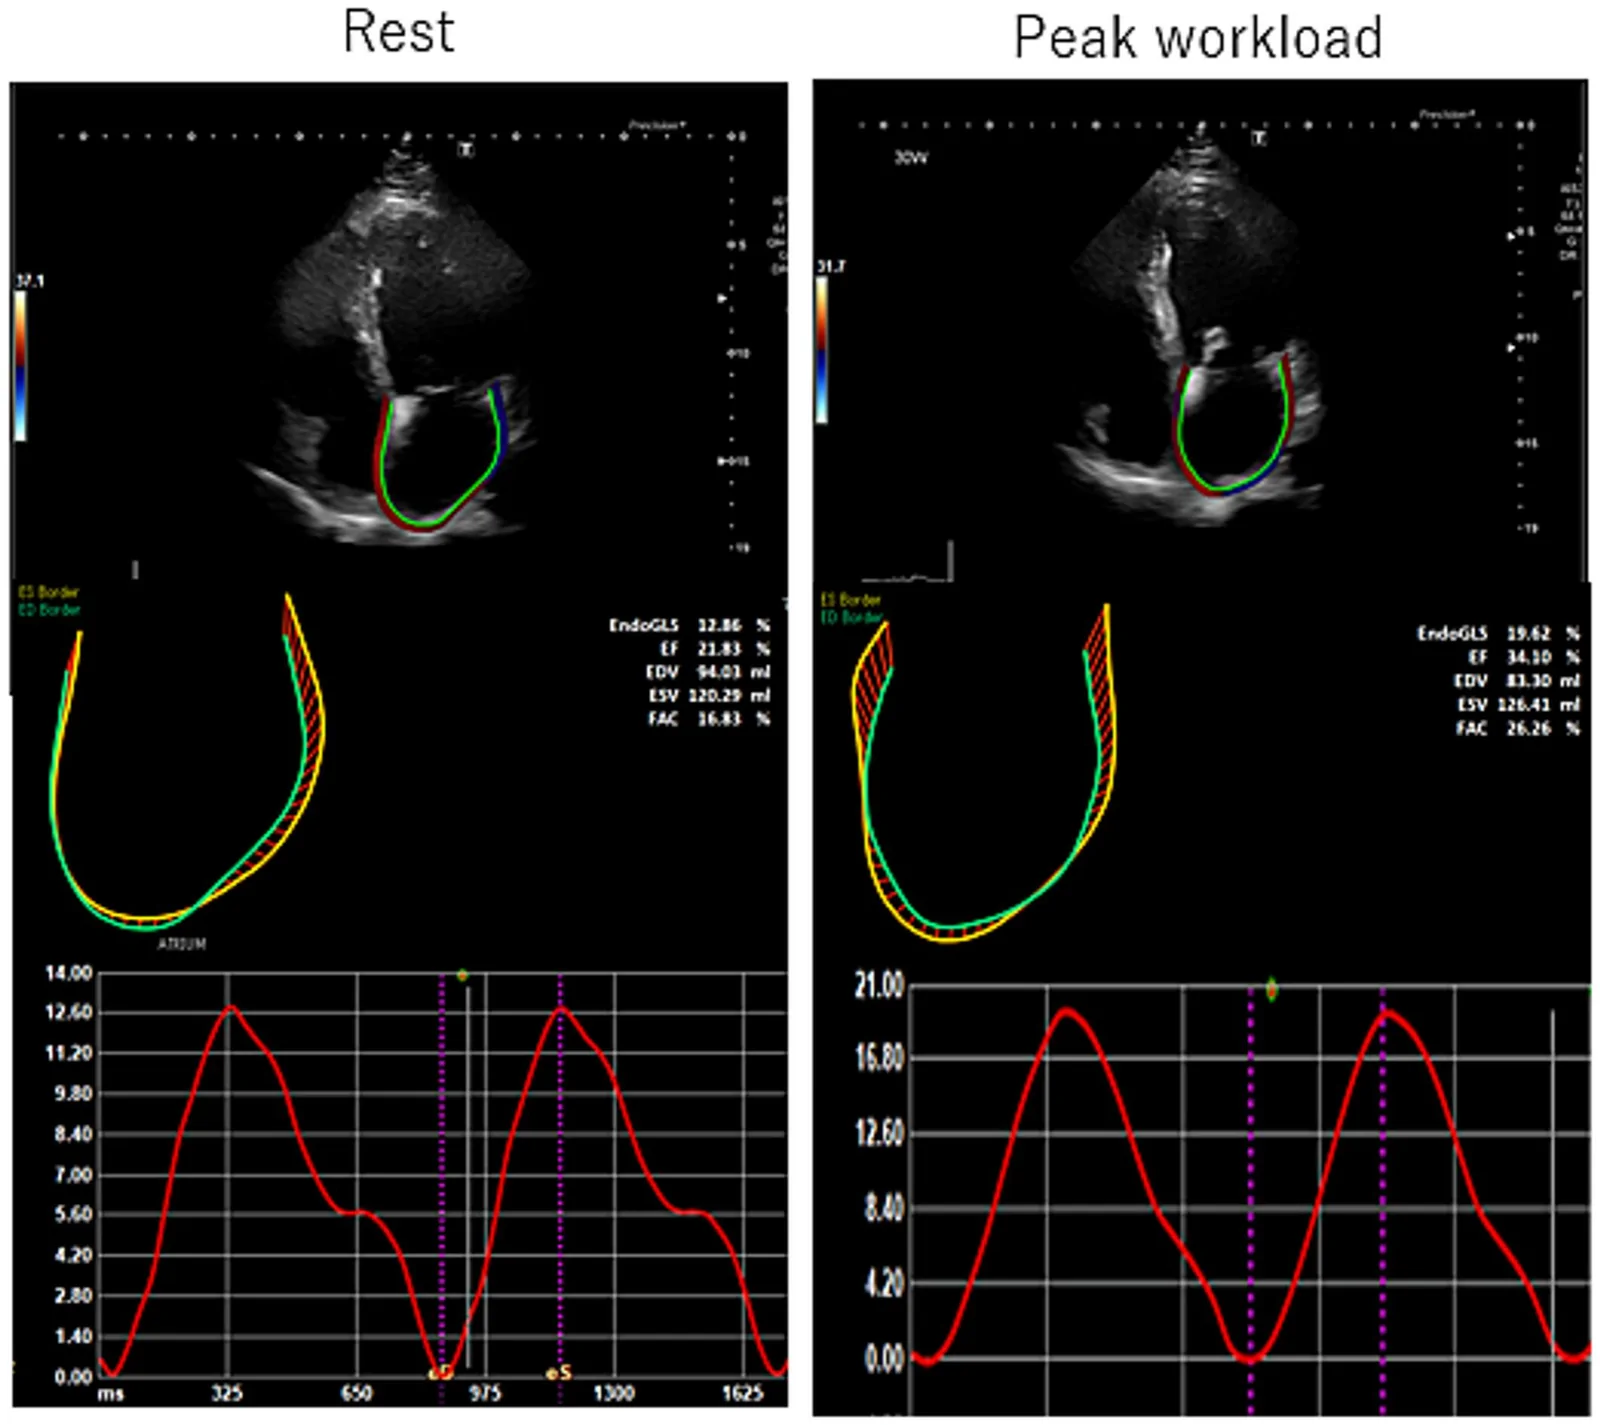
Representative images of LA reservoir strain at rest and peak workload. The speckle-tracking echocardiographic analysis of LA reservoir strain obtained from the apical four-chamber view. The left panel shows LA reservoir strain at rest of 12.9%. The right panel shows LA reservoir strain at peak workload of 19.6%. LA, left atrial.
